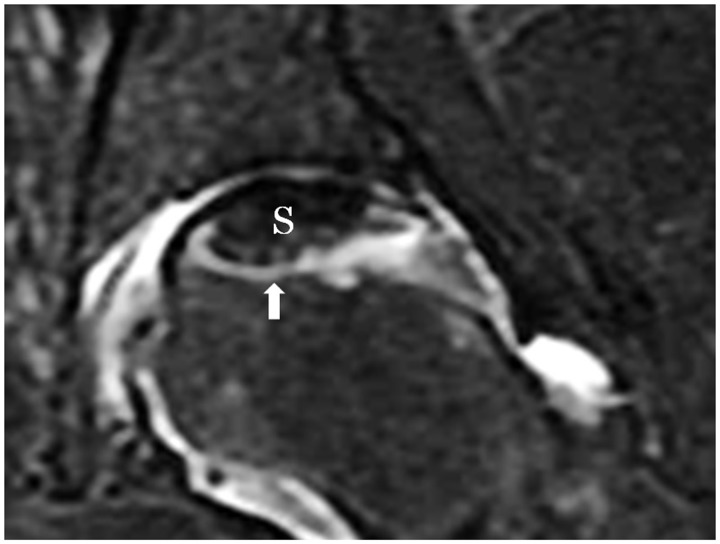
MRI T2-weighted fat suppression coronal image.
